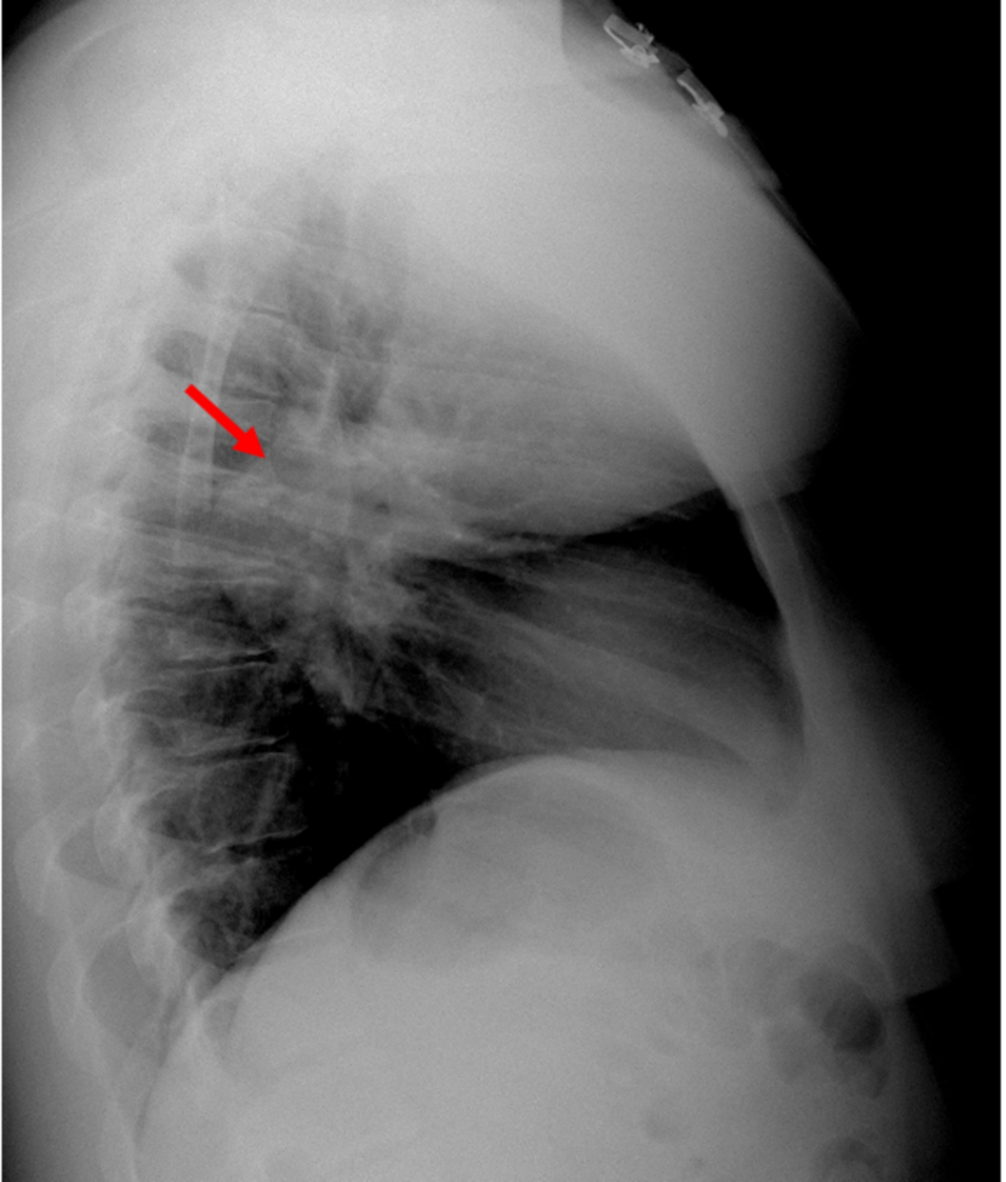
Chest X-ray (lateral view) showing a mass-like lesion in the posterior mediastinum (red arrow).
